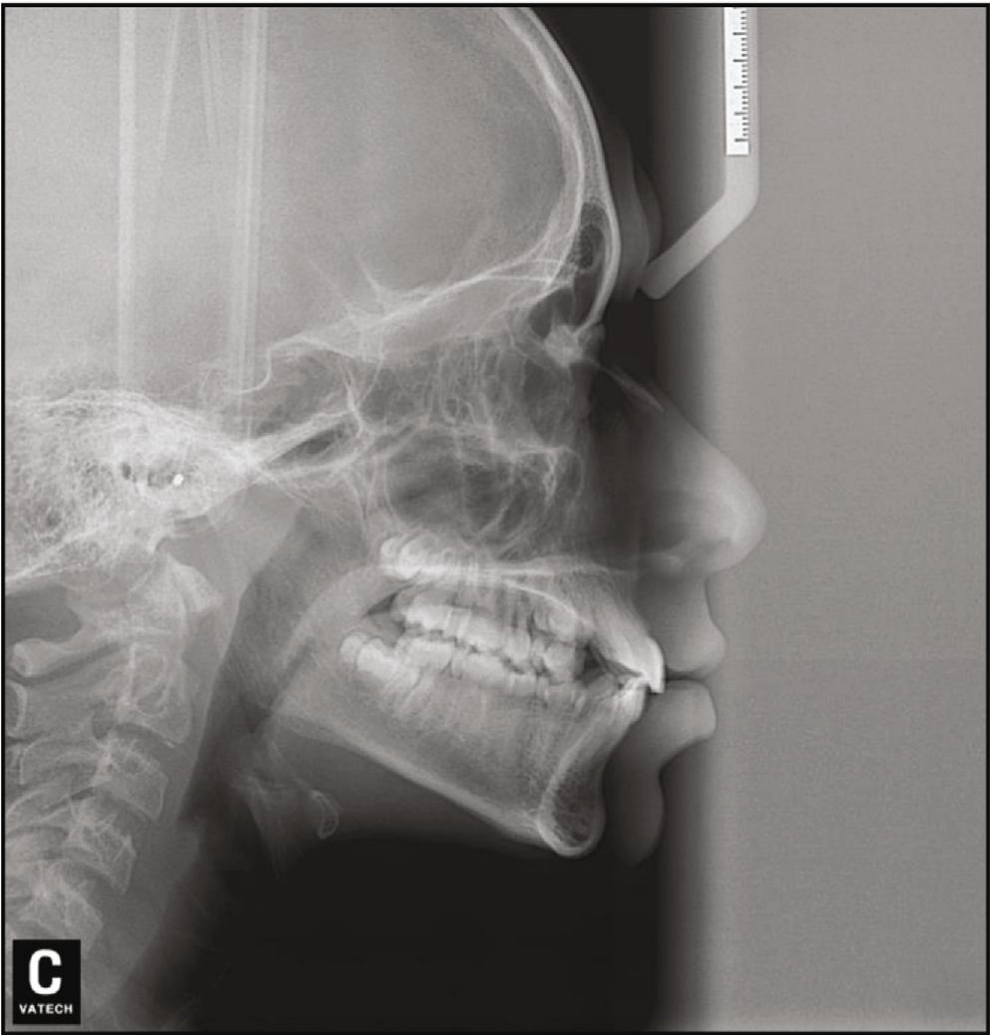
The lateral cephalometric radiograph.
